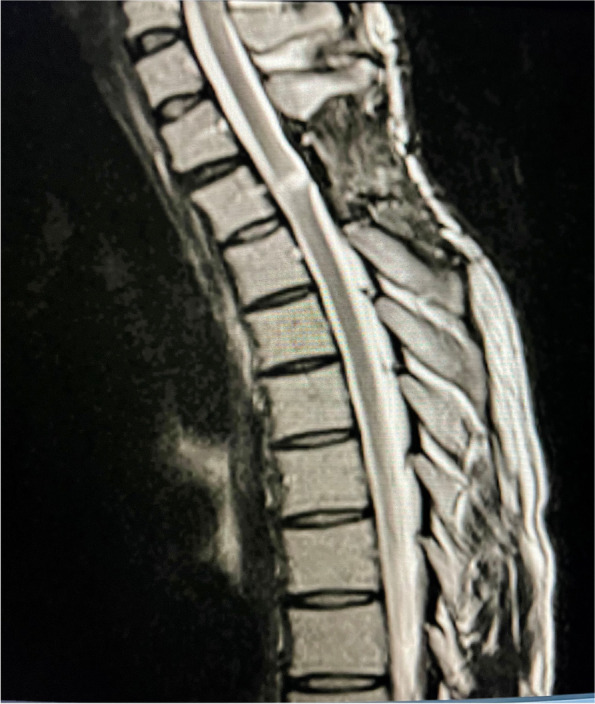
Three month Post-operative MRI T2 weighted lateral view demonstrating the high signal in the repaired cord and soft tissue changes
